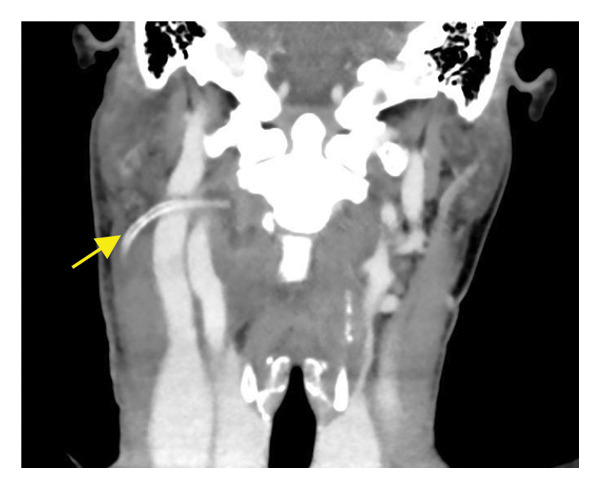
Postoperative contrast‐enhanced CT scan (coronal view) showing resolution of the previously described fluid collection and the drainage catheter in situ.
